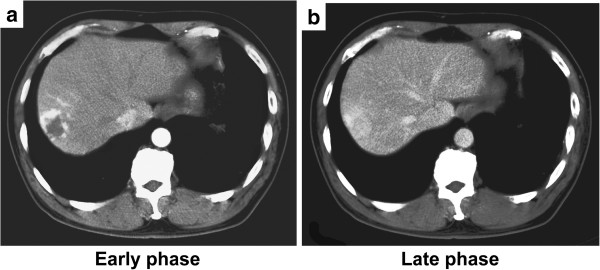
Two-phase dynamic incremental computed tomography (CT) of the lesion performed 10 years ago. a: In the early phase CT image, the lesion in segment 8 shows peripheral high attenuation. b: The late phase CT image demonstrates homogeneous high attenuation. These images demonstrate the progressive, centripetal contrast enhancement in a liver cavernous hemangioma.
